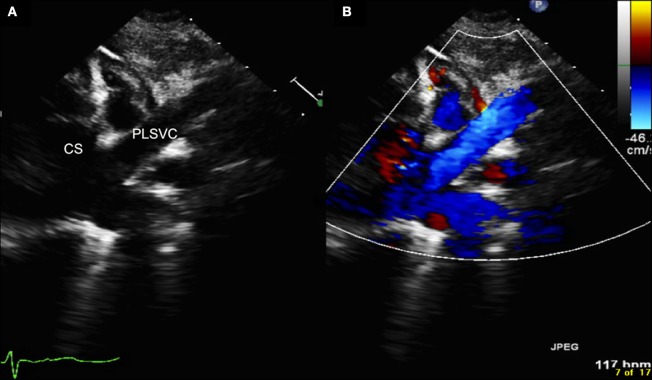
Echocardiography (A = normal, B = with color Doppler) showing the persistent left superior vena cava draining into the dilated coronary sinus. CS, coronary sinus; PLSVC, persistent left superior vena cava.
